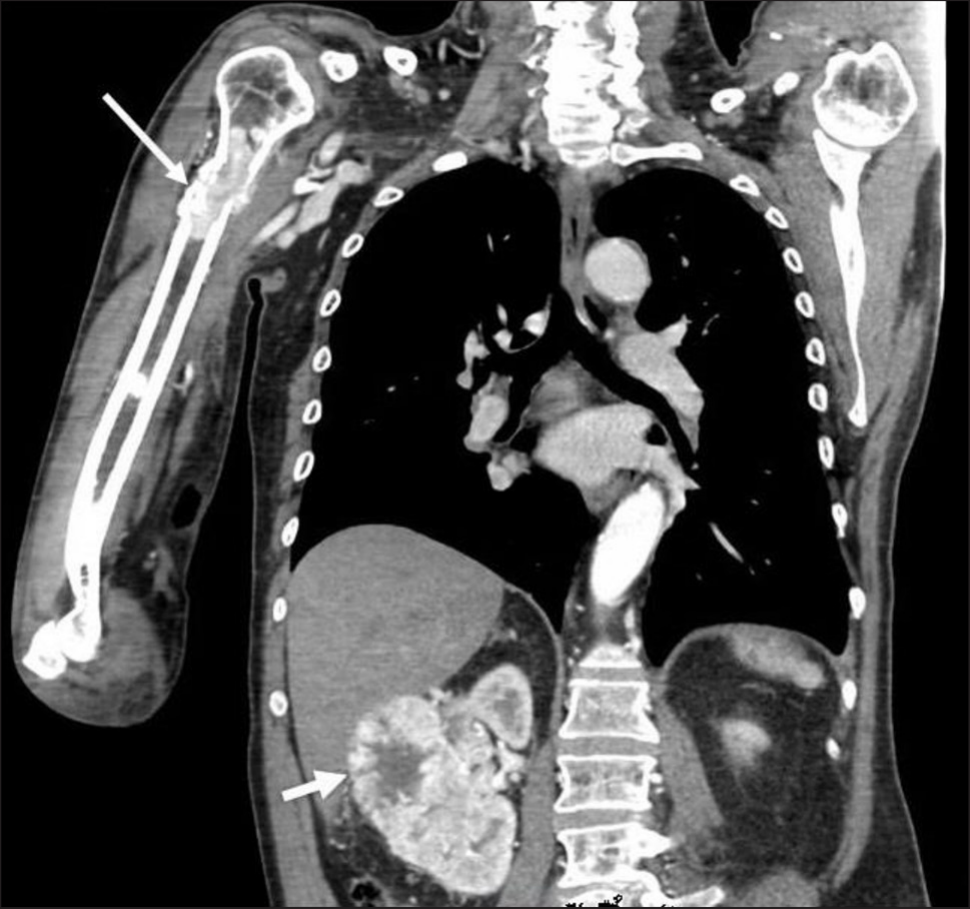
CT of thorax and abdomen: Enhancing lesion at the site of the fracture (long arrow). Exophytic mass at the mid and lower pole of the right kidney (short arrow).
